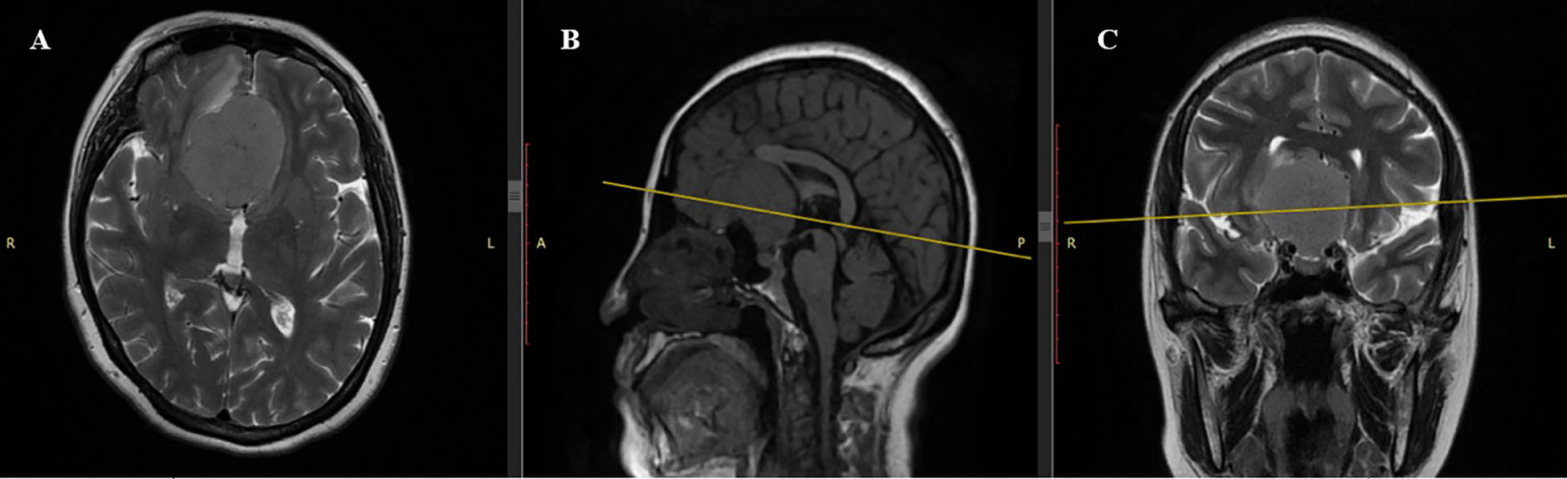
Preoperative MRI scan. (A) Axial T2-weighted sequence: Giant tuberculum sellae meningioma (4.81×4.85×4.26 cm) with well-demarcated margins and pronounced isointense signal, causing significant frontal lobe compression. (B) Sagittal T1-weighted sequence: Iso- to hypointense lesion with mass effect on basal structures, including the optic chiasm. (C) Coronal T2-weighted sequence: Isointense lesion occupying the olfactory groove, minimal peritumoral edema, and displacement of frontal lobes and optic chiasm.
